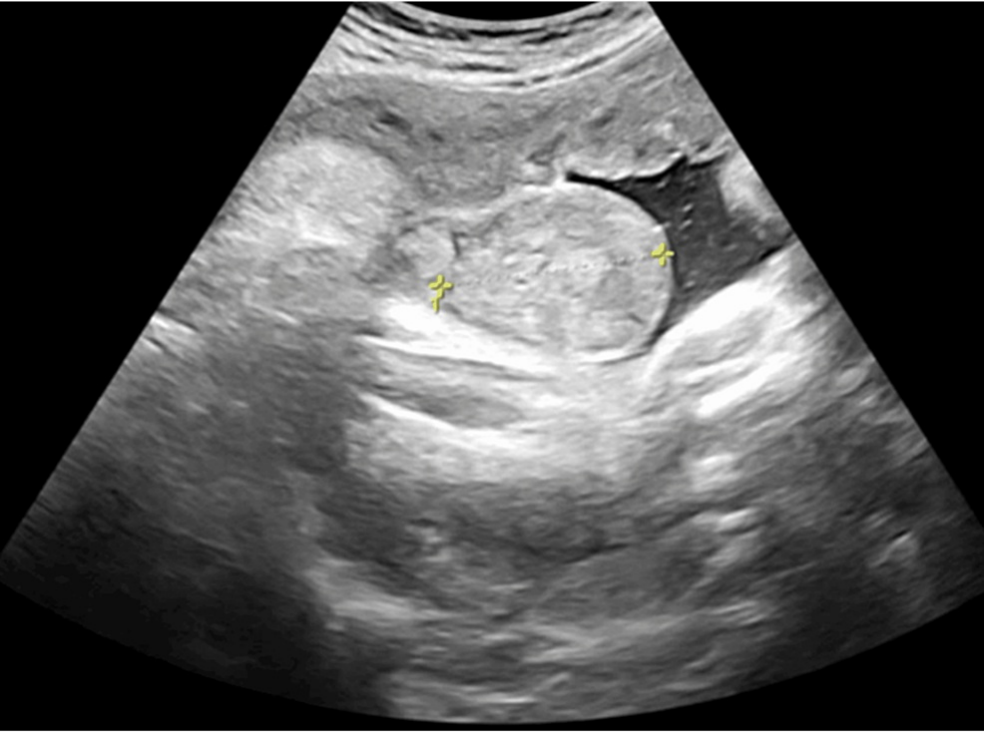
Transverse ultrasonographic image of fetal scrotum at 36 weeks gestational age, displaying right-sided enlargement (5.7 x 3.4 cm)
